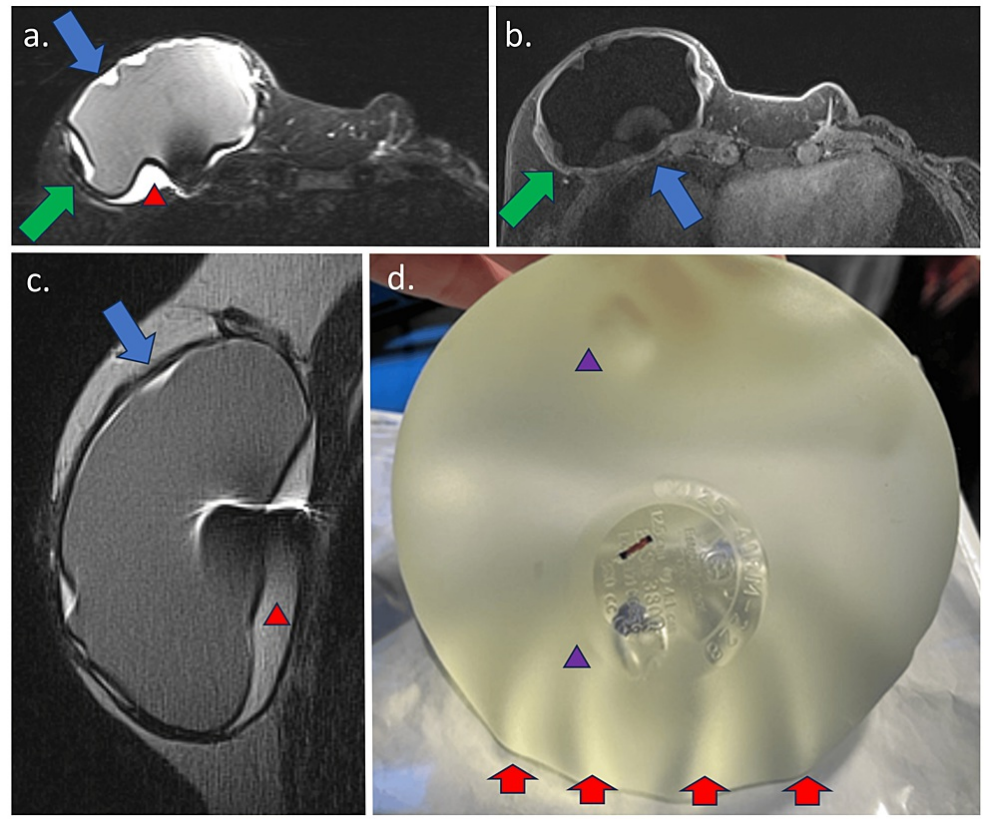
Breast MRI sequences showing MRI fibrous capsule classification Grade 4 and the macroscopy of the silicone implant in a 61-year-old patient with silicone implants for four years. The Baker classification was Grade 2. (a) Axial STIR sequence shows fibrous capsule thickening (blue arrow) associated with intracapsular mass (green arrow) and associated with intracapsular collection (red triangle); (b) T1 post-contrast sequence shows fibrous capsular thickening with a black-drop signal (blue arrow) with intracapsular focal thickening of the fibrous capsule associated with late contrast enhancement (green arrow); (c) The sagittal proton-density sequence shows the same findings associated with moderate intracapsular collection; (d) The implant's macroscopy shows permeability loss as droplets inside the silicone implants (purple triangle), with volume loss determining folds in the shell surface (red arrow). STIR: short tau inversion recovery Image Credit: Eduardo de Faria Castro Fleury
